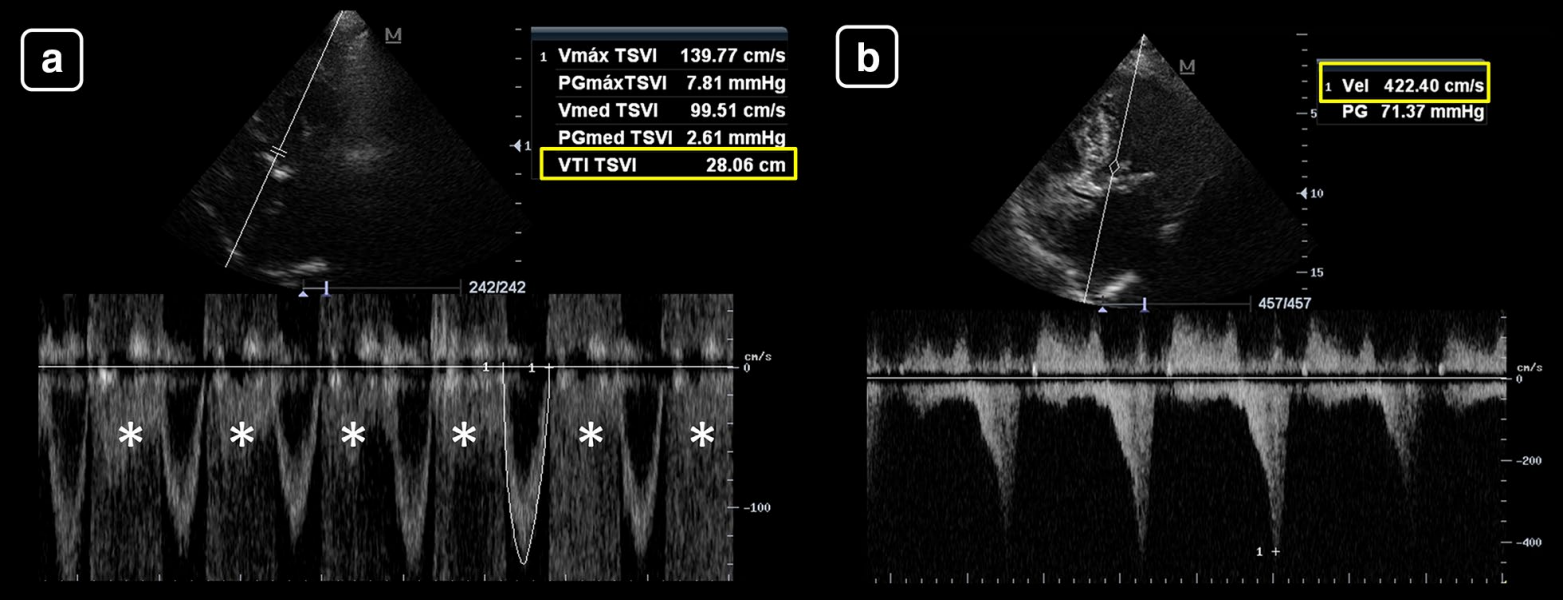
a Left ventricular outflow tract velocity–time integral (LVOT VTI) in severe aortic regurgitation. LVOT VTI showed high values (yellow box), overestimating the stroke volume. Asterisks: aliased spectral Doppler signal of aortic regurgitation. b LVOT VTI in dynamic LVOT obstruction, obtained with continuous wave Doppler (pulsed-wave Doppler waveforms were aliased). The spectral Doppler showed high blood flow peak velocities (4.2 m/s, yellow box) and a long time-to-peak signal. This situation can be seen in severe hypovolemia, hypertrophic cardiomyopathy, anterior myocardial infarctions with hypercontractile basal segments and in Takotsubo syndrome
